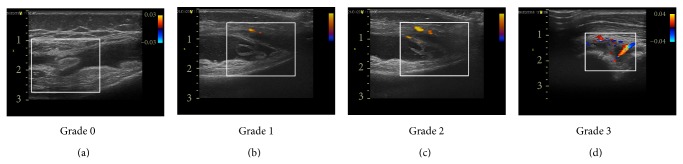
Ultrasound evaluation of joint vascularization scale using Power-Doppler Ultrasonography imaging. Examples of visualization of different degrees of joints inflammation and angiogenesis. Joint vascularization scale: grade 0 = no signs of vascularization, 1 = mild (presence of single/vessel dots), 2 = moderate (presence of confluent vessel dots in less than half of the synovial area), and 3 = marked (presence of confluent vessel dots in more than half of the synovial area).
